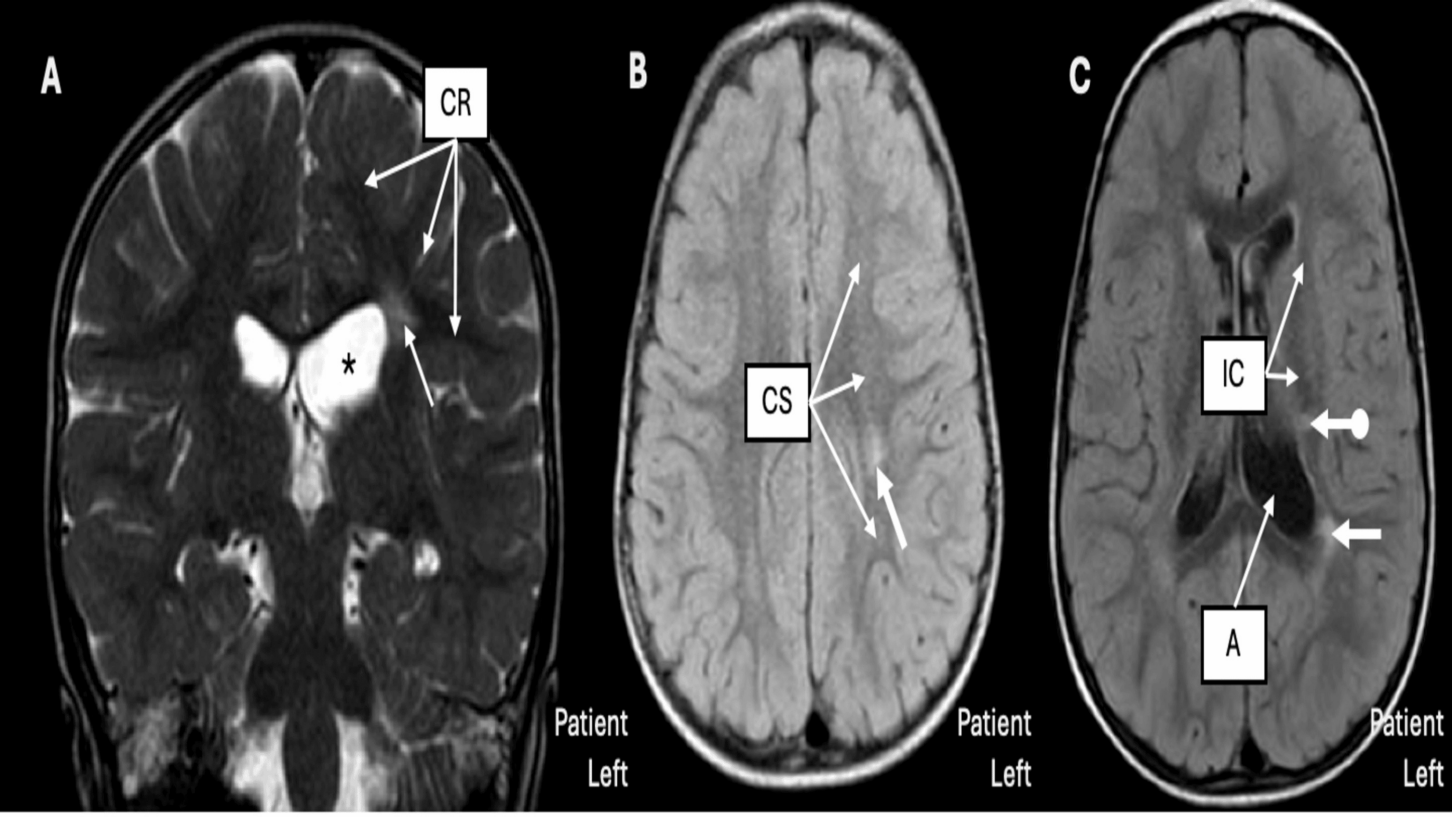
Brain MRI findings. Brain MRI demonstrating locations of the periventricular leukomalacia – radiographic evidence of early white matter damage. (A) Coronal T2 MRI showing the left corona radiata (CR), with the thick white arrow demonstrating the leukomalacia. Note the enlarged ventricle (*), confirming white matter loss in that region. (B) Axial fluid-attenuated inversion recovery showing the left centrum semiovale (CS) with the thick arrow showing the location of the leukomalacia. (C) Axial T1 showing both the internal capsule (IC) and ventricular atrium (A) with the periatrial leukomalacia (thick arrow) and the leukomalacia of the internal capsule (thick arrow with the ball tip).
